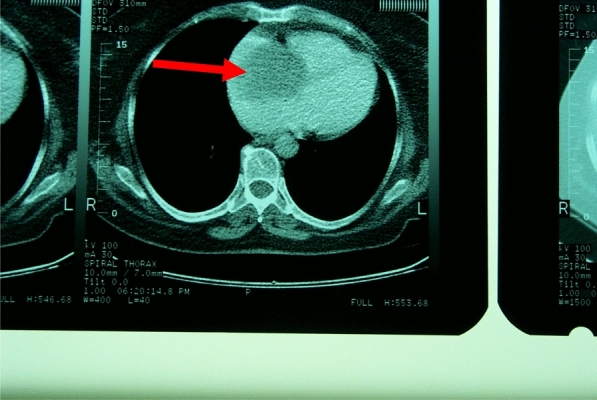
Appearance of the right atrial mass on computed tomography
